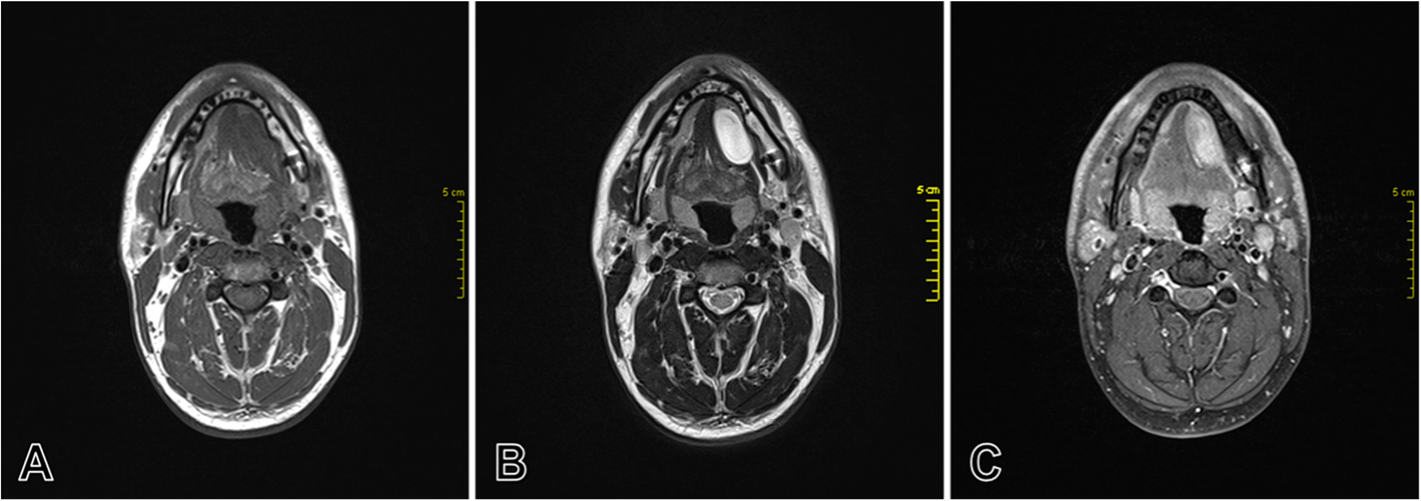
Axial MRI images of the floor of the mouth showing a mass on the left side. a, T1-weighted image shows low homogeneous signal of the mass. b, T2-weighted image shows high signal intensity of the mass. c, Gadolinium-enhanced T1-weighted Fast Sat image shows a heterogeneous enhancement of the signal intensity
